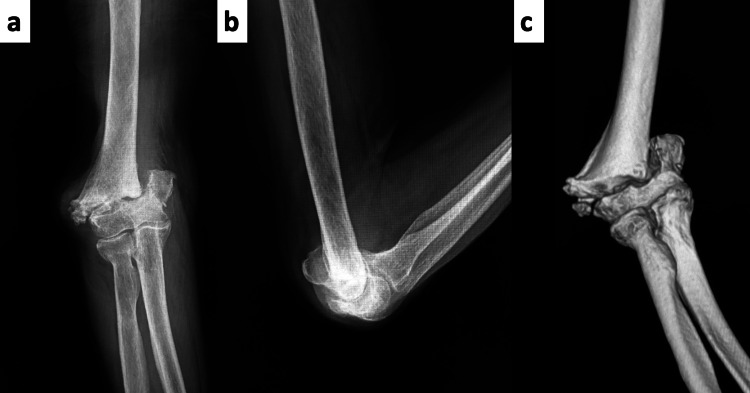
Preoperative Imaging (a) Anteroposterior (AP) view and (b) lateral view plain radiographs, along with (c) a preoperative 3D CT image, showed a nonunion of a transcondylar distal humeral fracture. While bone defects were significant, the articular surface remained intact.
